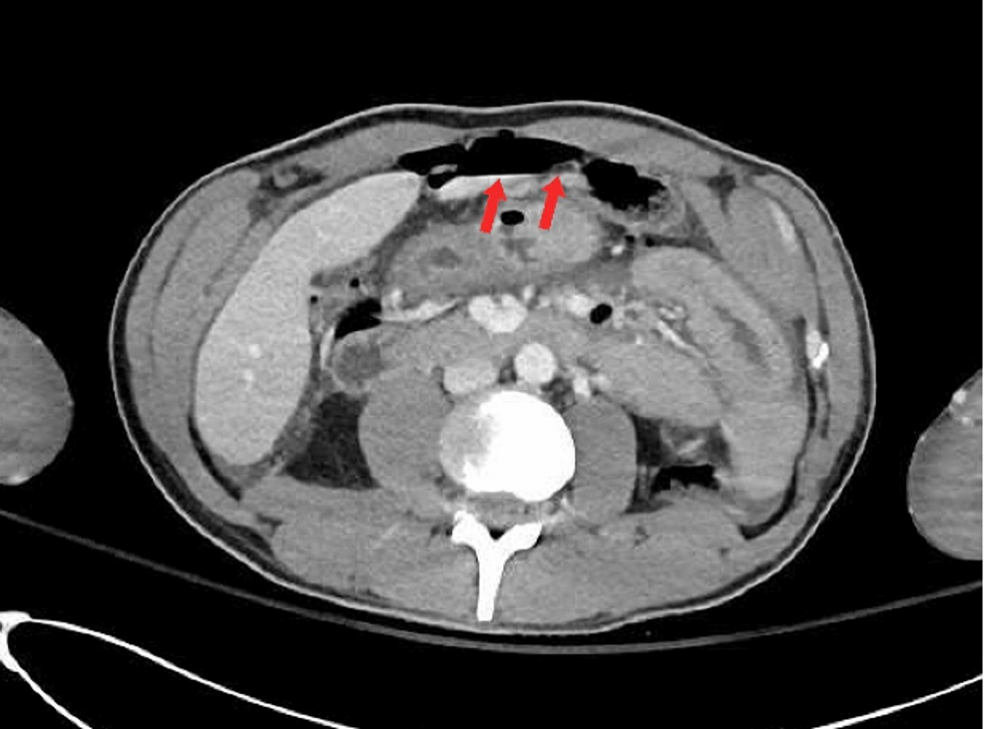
Abdominal CT scan exhibits extraluminal gas representing perforated viscus The red arrows in the figure point out the extraluminal gas, which appears black. CT: computed tomography
